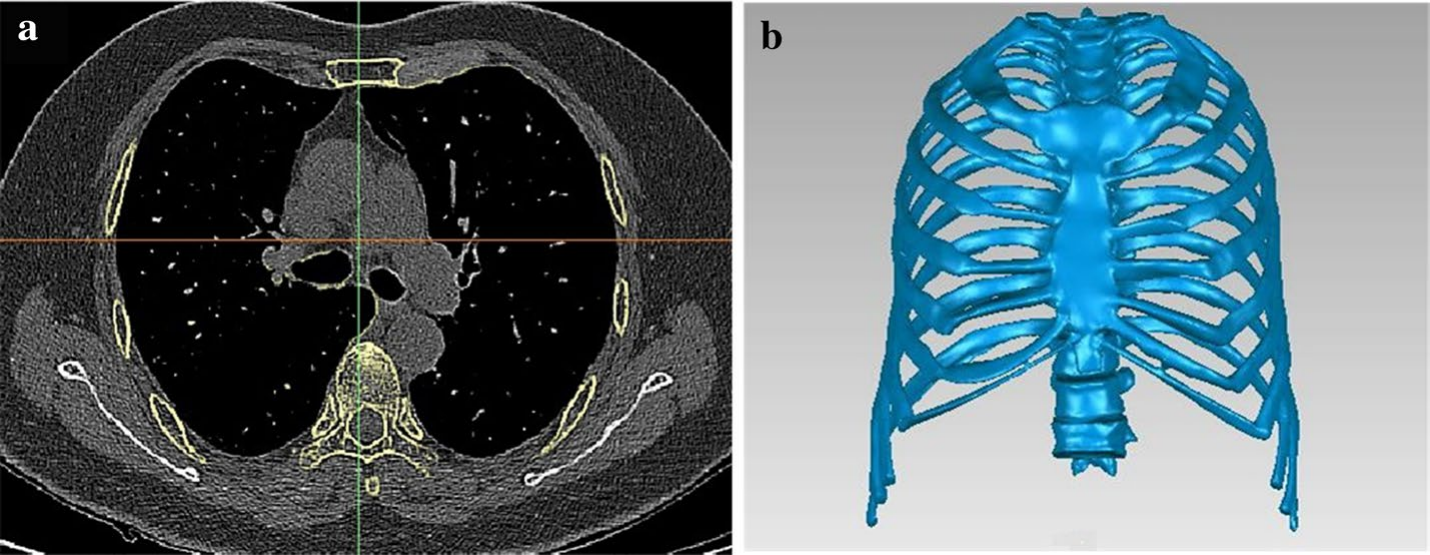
a 2D CT image of the thoracic tissue and b the final geometry
